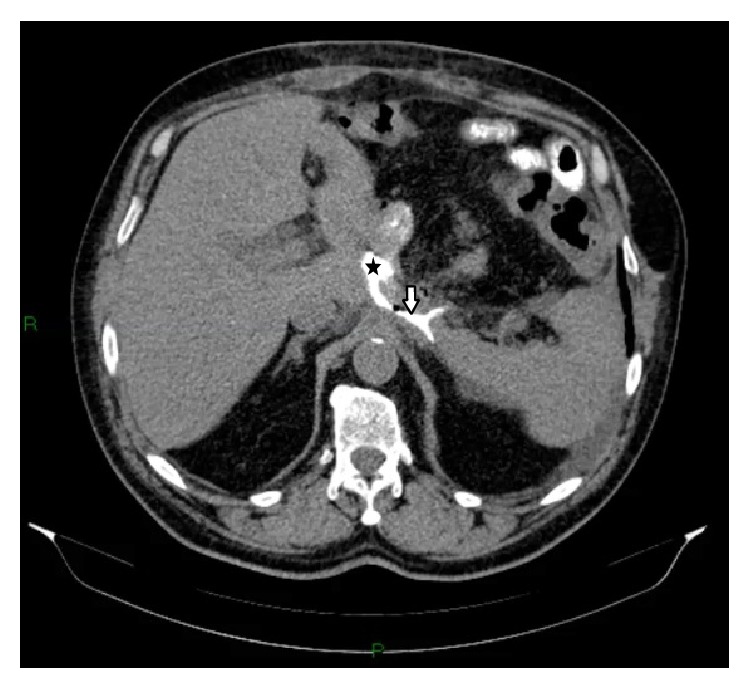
Axial contrast-enhanced abdominal computer tomography (CT): esophageal lumen (star); esophagojejunal anastomotic fistula (arrow).
